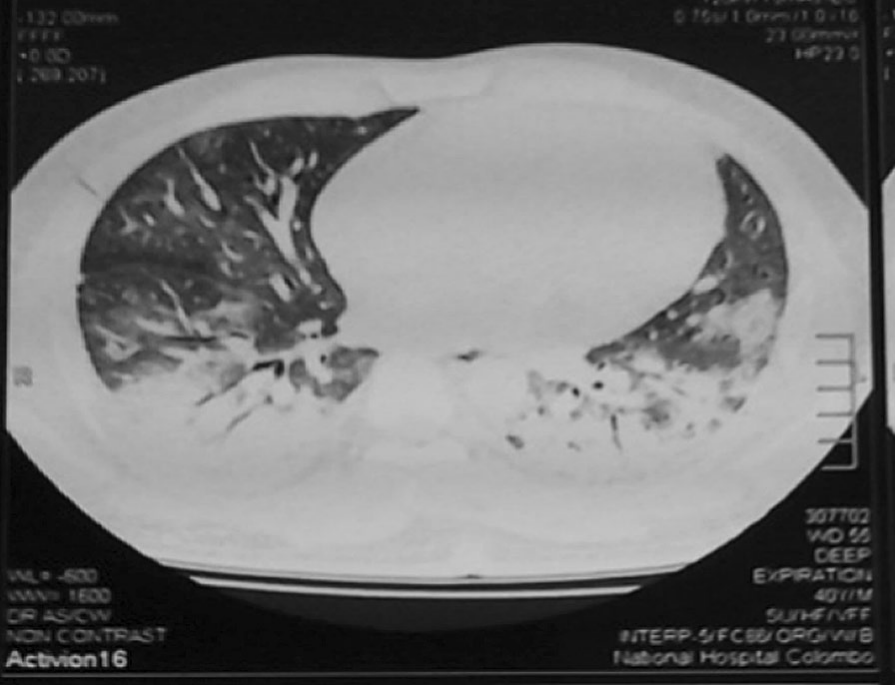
HRCT of the chest showing sub pleural patchy airspace consolidation and ground glass opacification with airbronchogram in bilateral basel regions with sparing of *upper* and *middle* zones, which was compatible with OP
